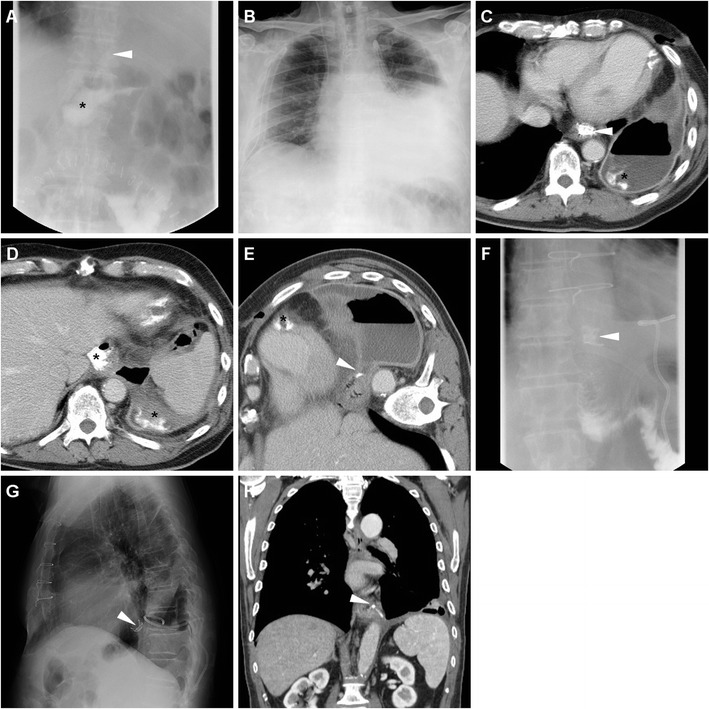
A 59-year-old male with cardial carcinoma underwent total gastrectomy. Early postoperative CF (A) showed patent EJS (arrowhead) with perianastomotic contrast leakage (*) directed medially. Bilateral pleural drainages were positioned to relieve minimal right pneumothorax and left hydropneumothorax (B). On 10th POD contrast-enhanced CT with oral contrast (C, D) confirmed ample left-sided hydropneumothorax with enhancing pleural surface. Extraluminal contrast leakage (*) was seen both medially to the EJS (arrowheads) and dependent in the left pleural empyema. Repeated CT obtained in the right lateral decubitus position (E) before CT-guided pleural drainage confirmed anastomotic fistula from the EJS (arrowhead) to the hydropneumothorax, which was treated by endoscopic clipping (arrowhead in F); note pleural pigtail drainage
